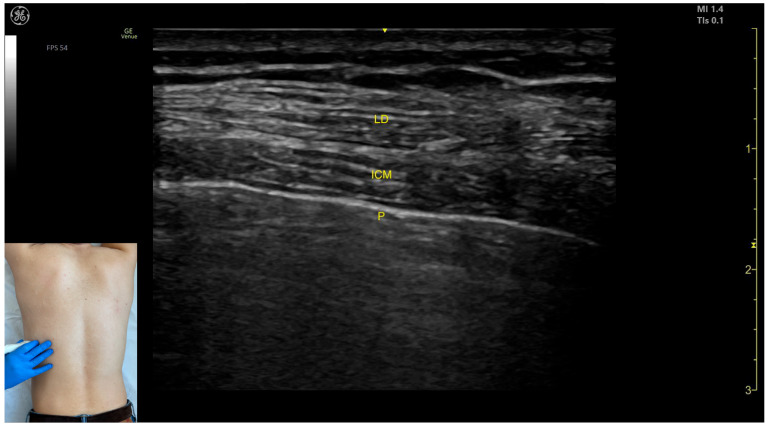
Ultrasound anatomy of the latissimus dorsi (LD) with key landmarks: LD—latissimus dorsi; ICM—intercostal muscle; and P—pleura.
